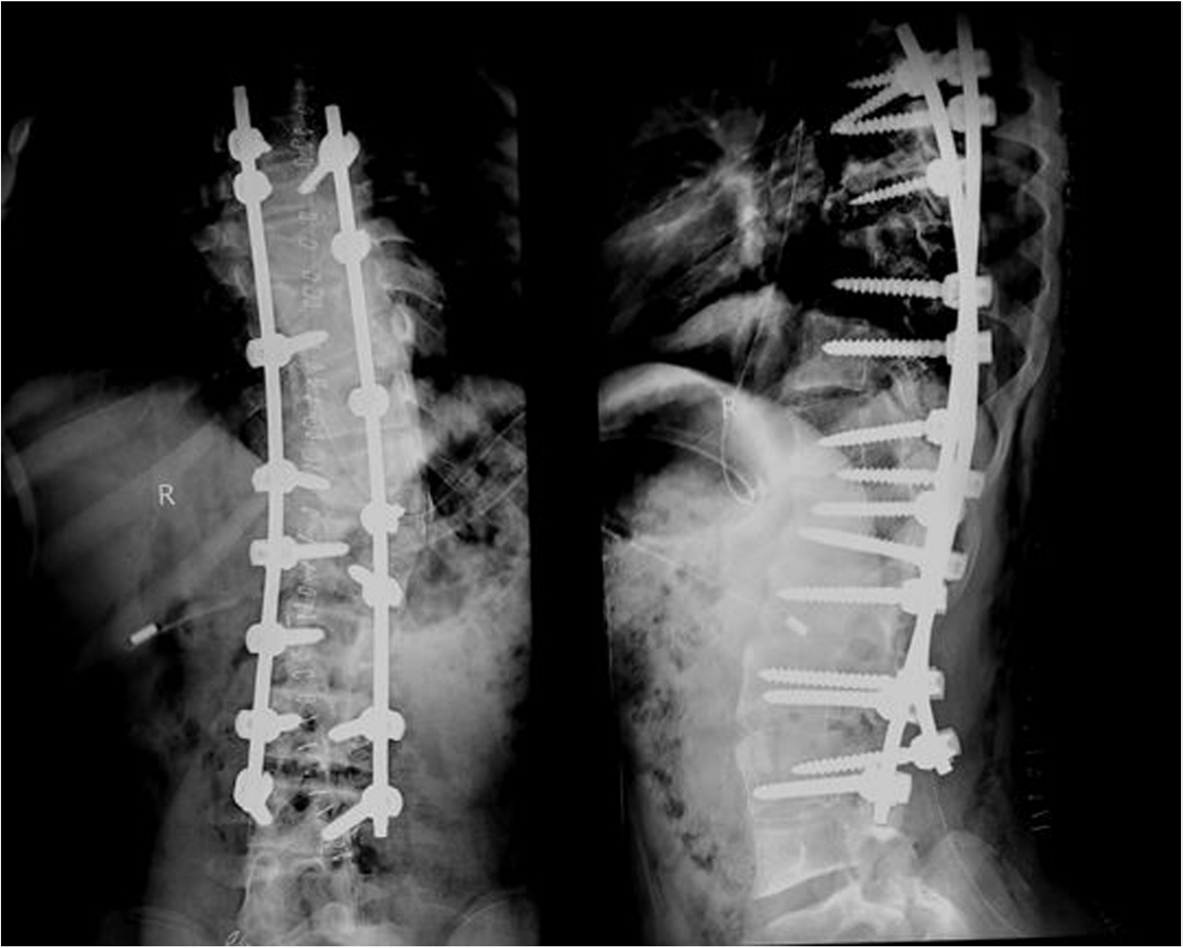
Immediate post-operative radiograph after deformity correction and instrumented fusion from D4 to L4 levels, showing good curve correction of 68.5% from pre-operative measurements. Posterior instrumented fusion with contoured rods and pedicle screws are visible
